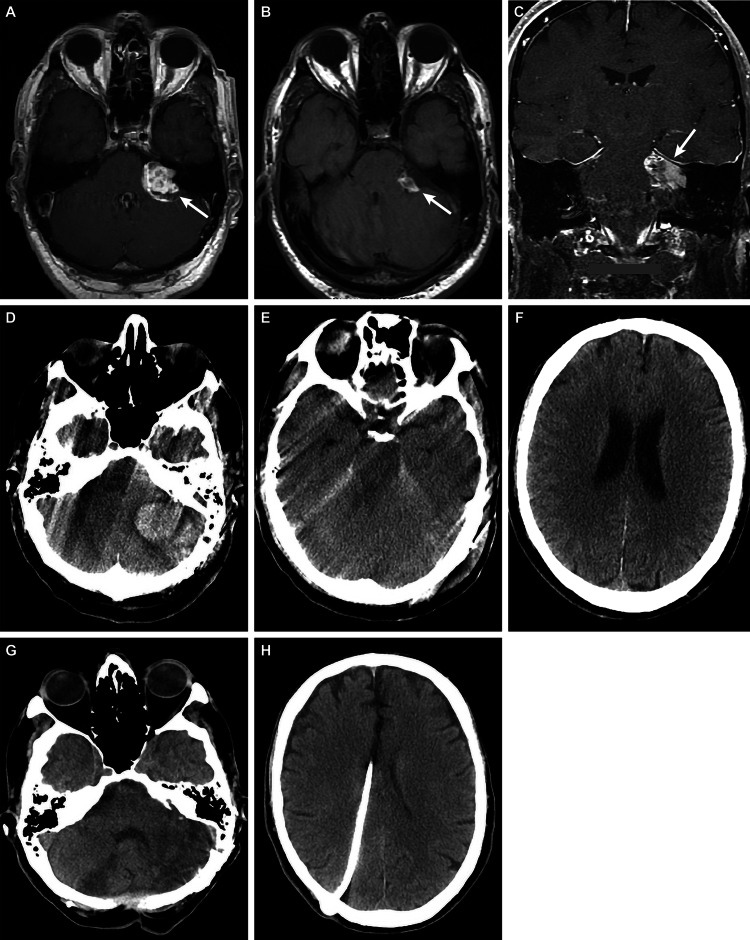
Case 2 – a 65-year-old man who presented with left-sided hearing loss (A) Preoperative axial T1-weighted MRI showed a 3.0 × 2.6-cm nonhomogeneous lesion in the cerebellopontine (CP) angle (arrow), suggestive of a vestibular schwannoma on the left labyrinth, compressing the brainstem. Postoperative (B) axial and (C) contrast-enhanced coronal T1-weighted MRI showed postoperative changes consistent with left retrosigmoid craniotomy for debulking of the left vestibular schwannoma. Fat packing could be seen (arrows). (D-F) Axial CT on postoperative day three showed postoperative changes and revealed (D) a new left posterior fossa hemorrhage measuring 4.4 × 3.1 cm, which caused cerebellar swelling, brainstem compression, fourth ventricle obstruction, (E) loss of basal cisterns, and (F) hydrocephalus. Axial CT, after multiple surgical interventions following a stroke, demonstrating (G) cerebellum resection and (H) ventriculoperitoneal shunt placement in the left lateral ventricle MRI: magnetic resonance imaging; CT: computed tomography Used with permission from Barrow Neurological Institute, Phoenix, Arizona
